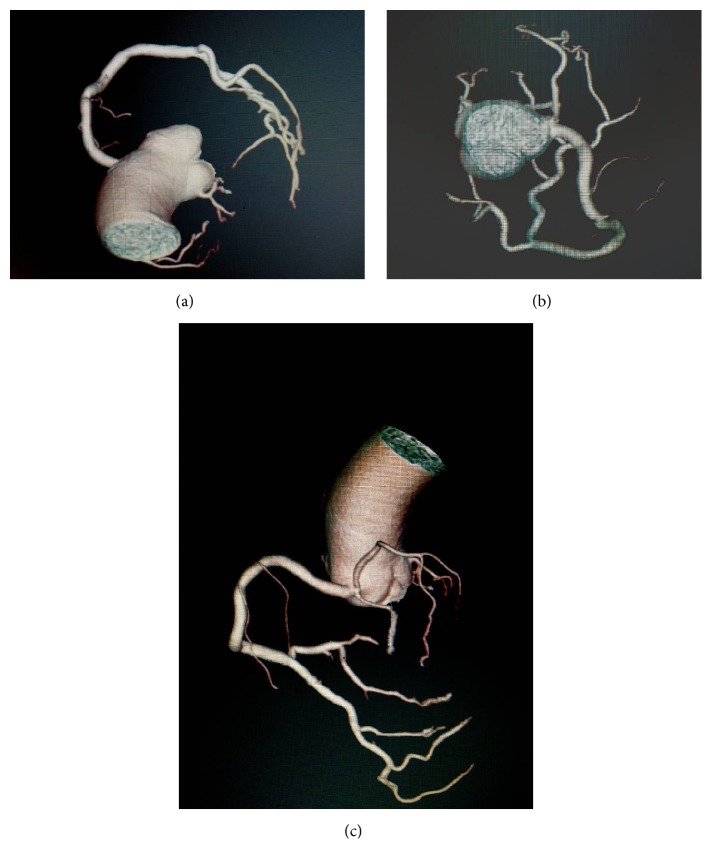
(a, b, and c) CT image of coronary artery.
